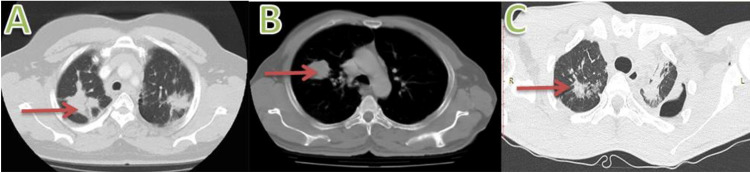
CT scan of the patients. A: Non-small cell carcinoma in the lung. B: Metastatic carcinoma in the lung. C: Endobronchial tuberculosis.
